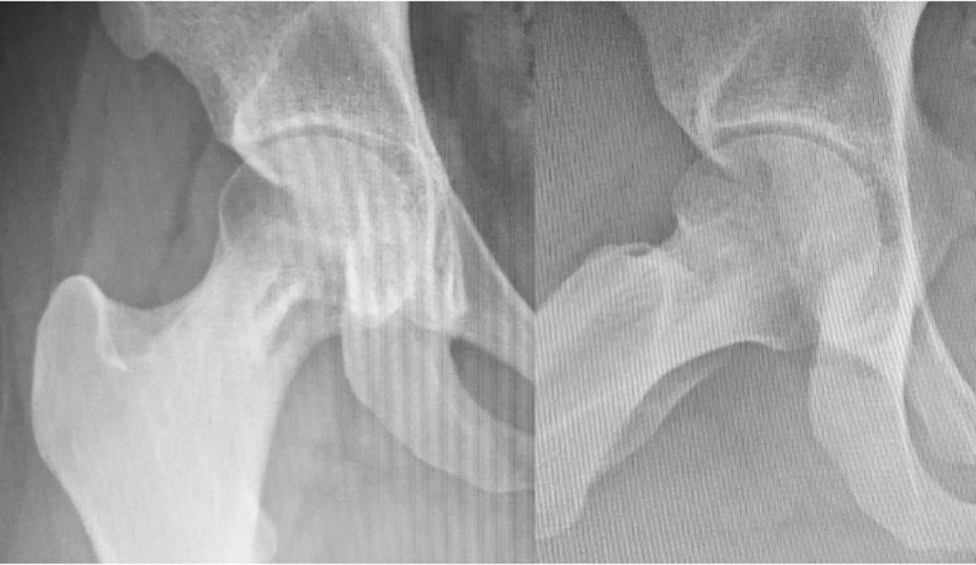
Radiograph taken 180 days after surgery demonstrating bone formation on the previous lesion.
